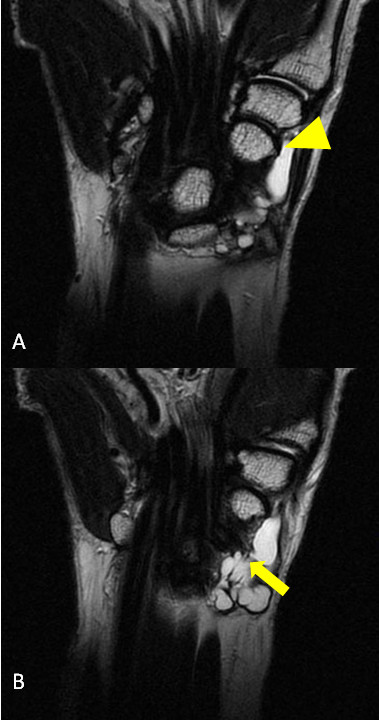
Magnetic resonance imaging results. (A, B) T2-weighted fat-suppressed magnetic resonance image showing origination of the multicystic lesion (arrow) from the Scaphotrapezial joint (A; arrow head) and expansion to the distal forearm beyond the wrist (B).
